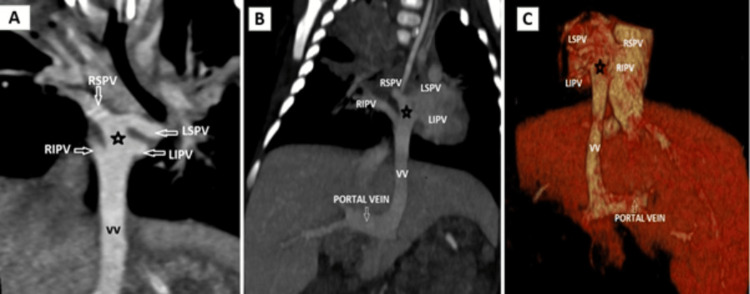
Infracardiac TAPVC (A) cardiac CT coronal MIP image showing the confluence of all pulmonary veins (black star) joining to form a common descending VV and (B and C) post-contrast coronal MIP and 3D VR images showing the vertical vein coursing through the diaphragmatic hiatus and draining into the dilated main portal vein TAPVC: total anomalous pulmonary venous connection, MIP: maximum intensity projection, CT: computed tomography, VV: vertical vein, VR: volume rendered, RSPV: right superior pulmonary vein, RIPV: right inferior pulmonary vein, LSPV: left superior pulmonary vein, LIPV: left inferior pulmonary vein
